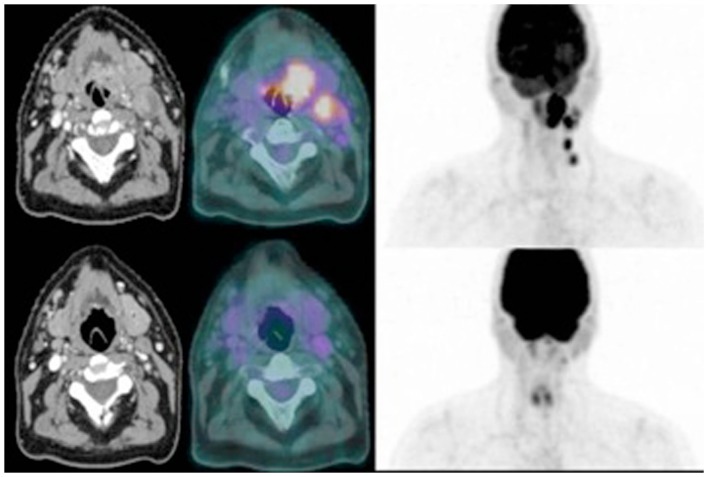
Comparative axial images from contrast-enhanced CT (left), and ^18^F-FDG-PET/CT (right) for a patient with T2N2b SCC tonsil before (top) and after (bottom) induction chemotherapy.
